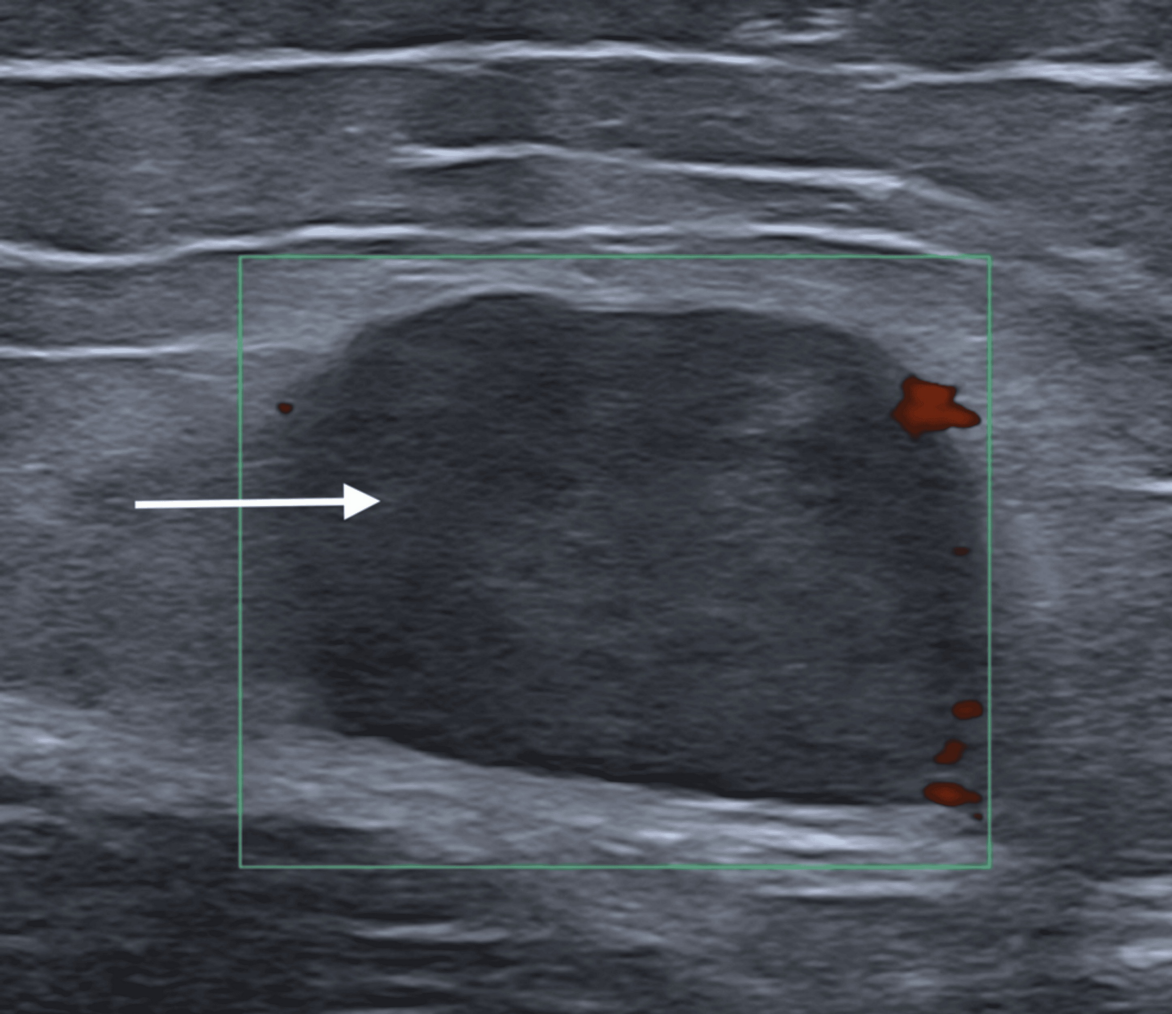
Ultrasound of the left hip shows a well-defined, oval, hypoechoic subcutaneous soft-tissue mass overlying and adjacent to the tensor fasciae latae (white arrow). Color Doppler shows no meaningful internal vascularity.
